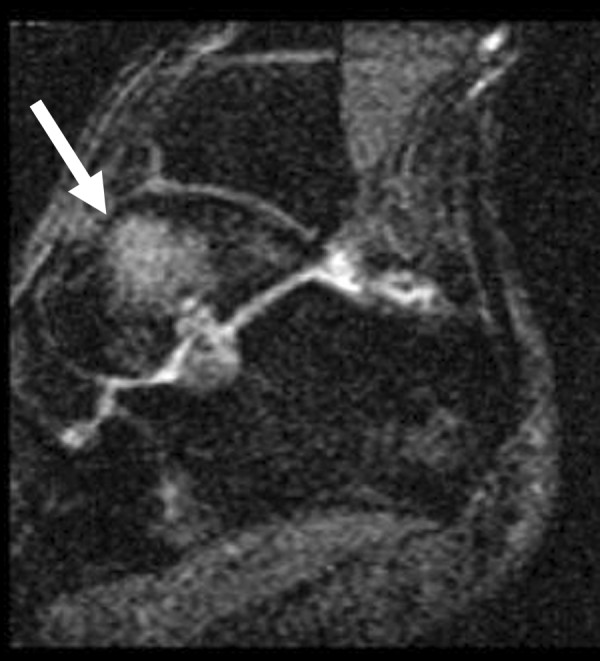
Typical appearance of talar bone marrow edema observed on MR images of nine out of twelve ankles of symptomatic professional ballet dancers: Sagittal STIR (TR/TE = 1800/24) image of a 24-year-old male ballet dancer shows associated edema-type high signal within the talus (arrow).
